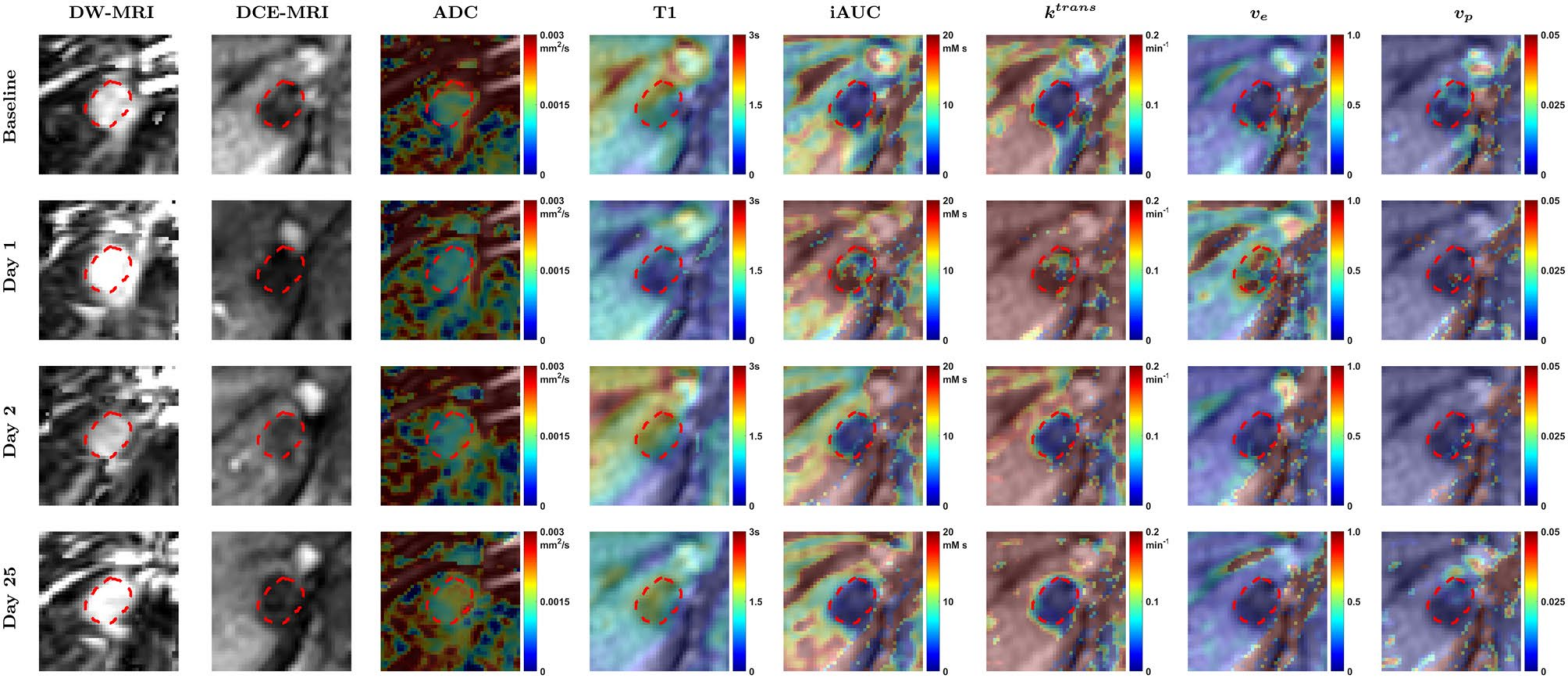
DW-MRI (b = 0 s/mm^2^), DCE-MRI images (2nd time point after AIF peak), and parameter maps per visit of a metastatic colorectal cancer tumor located in the liver of patient 102–003-105 (tumor 2). Contour annotation of tumor is shown as dashed red line. After PEGPH20 (Day 1) a decrease in ADC and T1, and an increase in iAUC, k^trans^, and v_e_, within the tumor are observed. An increase in v_p_ is observed in few pixels.
